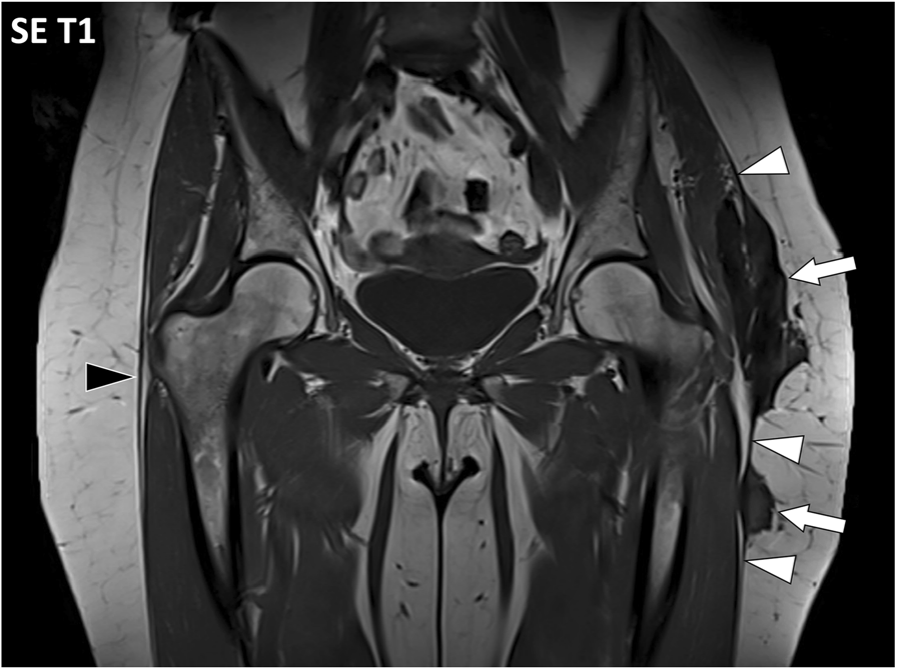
Coronal SE T1w image of the pelvis of a 52-year-old female with a desmoid tumor in the left hip area. MRI demonstrates low signal intensity masses (arrows) in continuity with the iliotibial tract and the deep peripheral fascia with typical aspect described as “fascia tail sign” (white arrowheads). The right iliotibial tract and deep peripheral fasciae are normal (black arrowhead)
